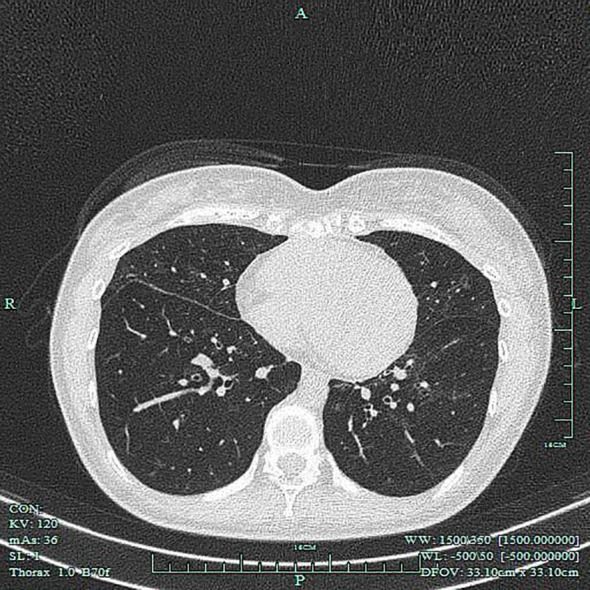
Follow-up chest CT scan at 2 months. The image reveals significant resolution of the previously noted pulmonary exudates, correlating with clinical improvement after therapy.
